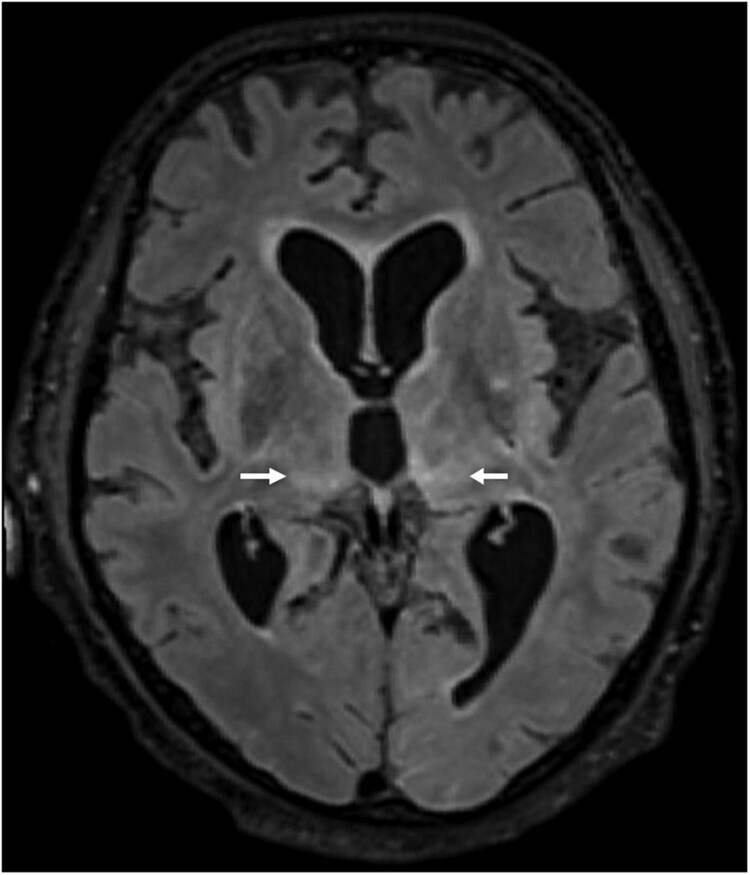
Cranial magnetic resonance imaging of case 2. In this case of a confirmed BoDV-1 encephalitis, widespread increase of signal intensity, especially of the thalami (arrows) is detected. Transversal T2-weighted fluid attenuated image (FLAIR).
